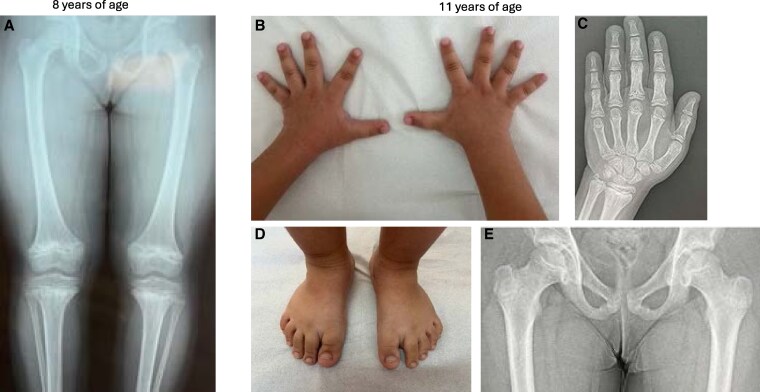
Lower limb radiograph at 8 years shows metaphyseal widening and irregularity at the knees, both in distal femurs and proximal tibiae, whereas the proximal femurs show normal femoral necks and metaphyses; epiphyses are normal in shape (A). At 11 years, hands show short fingers (B). Hand radiograph shows short metacarpals and phalanges with metaphyseal flaring and irregularity, cone-shaped phalangeal epiphyses (4th and 5th finger proximal phalanx) (C). Bone age development is uneven, with absent radial sesamoid bone but premature closure of some distal phalangeal growth plates (C). Feet are short and broad with short digits (D). Hip radiograph shows very mild metaphyseal irregularity and normal secondary ossification center (E).
